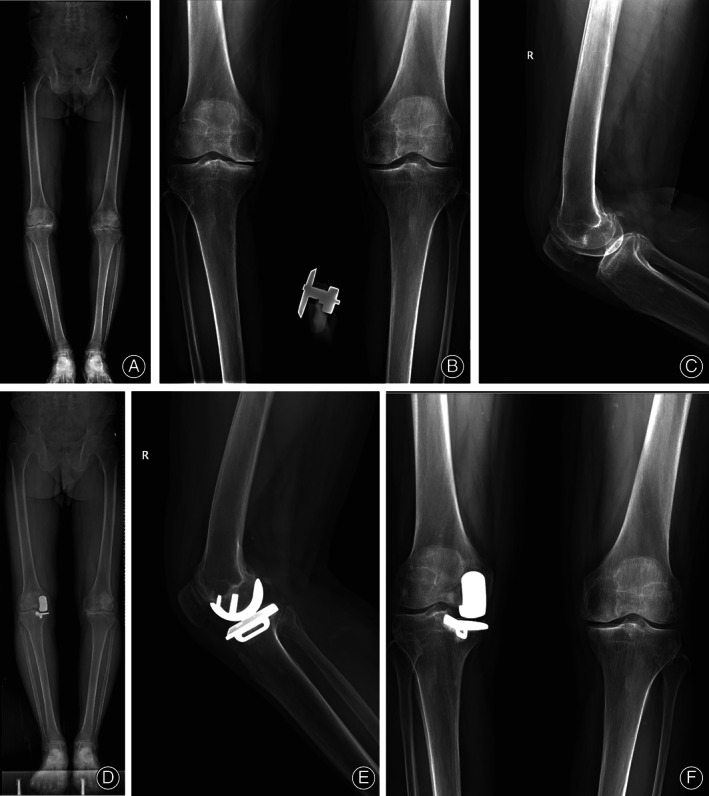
Male, 65 years old, diagnosed as AMOA and underwent UKA, showed a perfect radiographic result 1 year after surgery. (A) Lower limb length of X‐ray preoperatively. (B) Anteroposterior X‐ray preoperatively. (C) Lateral X‐ray preoperatively. (D) Lower limb length of X‐ray postoperatively. (E) Anteroposterior X‐ray postoperatively. (F) Lateral X‐ray postoperatively.
